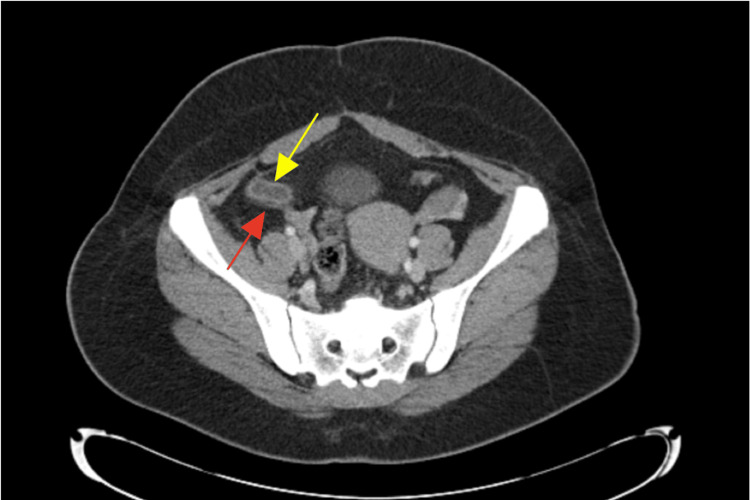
Transverse CT of abdomen on postoperative day eight Yellow arrow: Appendiceal wall thickening without an overt source of obstruction; Red arrow: Fat stranding surrounding the appendix signifying an inflammatory reaction
